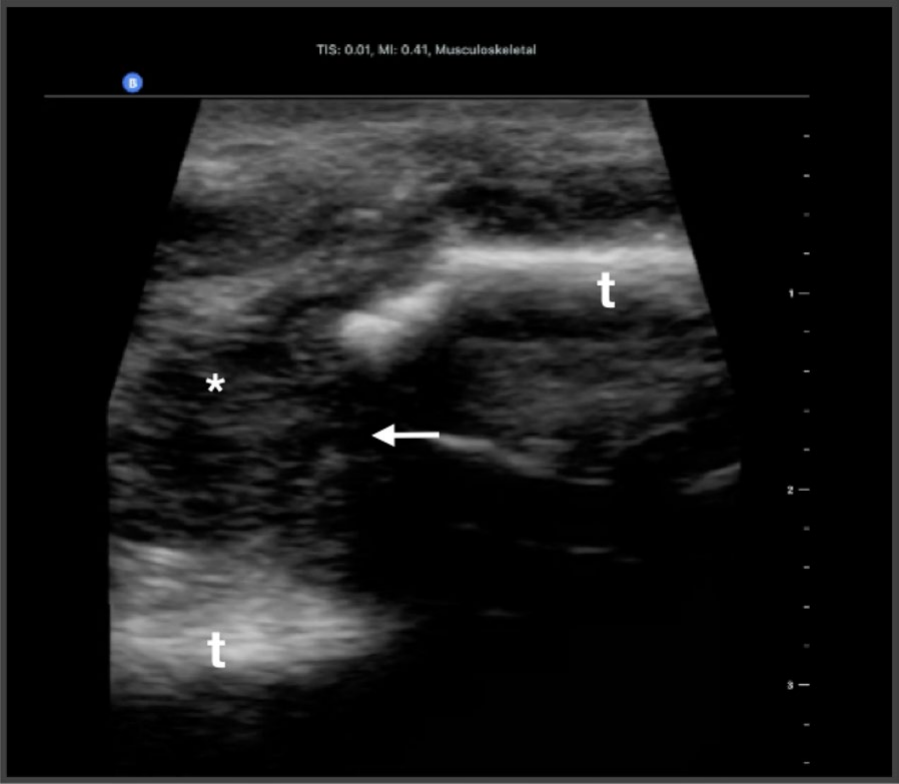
Musculoskeletal ultrasound of a young male patient with blunt trauma demonstrating two separate sections of tibial cortex (t), with displacement (arrows). Note the associated fracture hematoma visualized (*)
